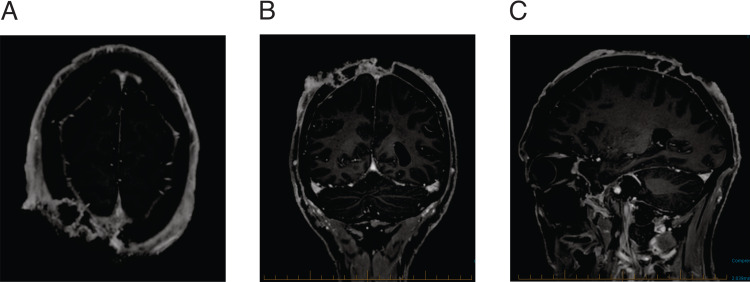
T1 MR image at presentation. (A) Axial image, (B) coronal image, and (C) sagittal image
